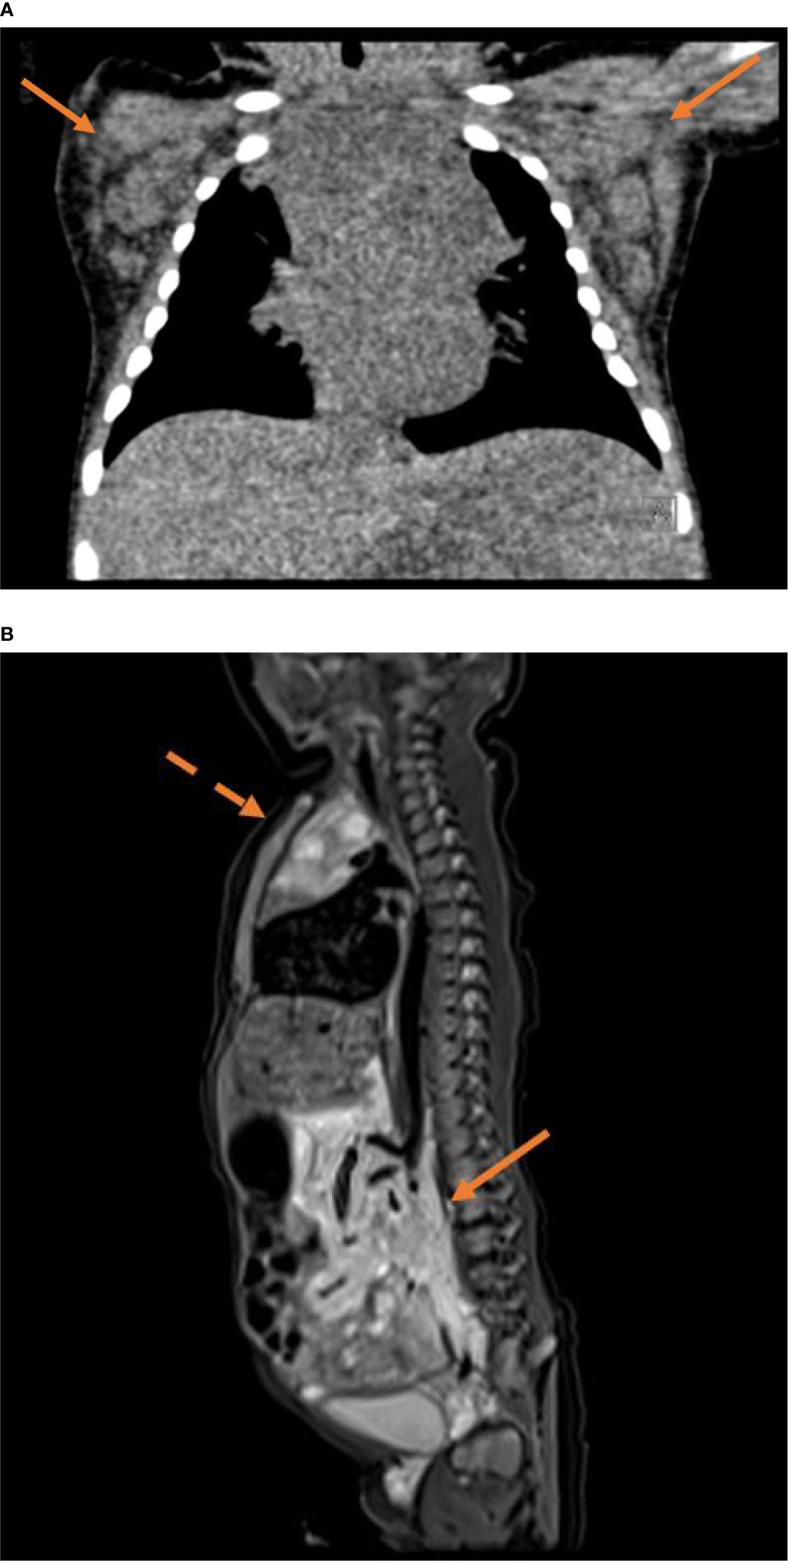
(A) Noncontrast CT (80kV, 48mAs, FOV 170x130mm) with coronal reconstruction using a soft tissue kernel shows a distinct bilateral axillary lymphadenopathy (arrows) and a prominent inhomogeneous thymus. (B) Sagittal T2 STIR sequence of a whole body MRI (TE 33ms, TR 3800ms, FOV 300x300mm, matrix 256x256px) shows bulky hyperintense soft tissue masses surrounding the aortocaval and mesenteric vasculature (solid arrow). The enlarged thymus features multiple jagged-edged cystic lesions (dotted arrow).
